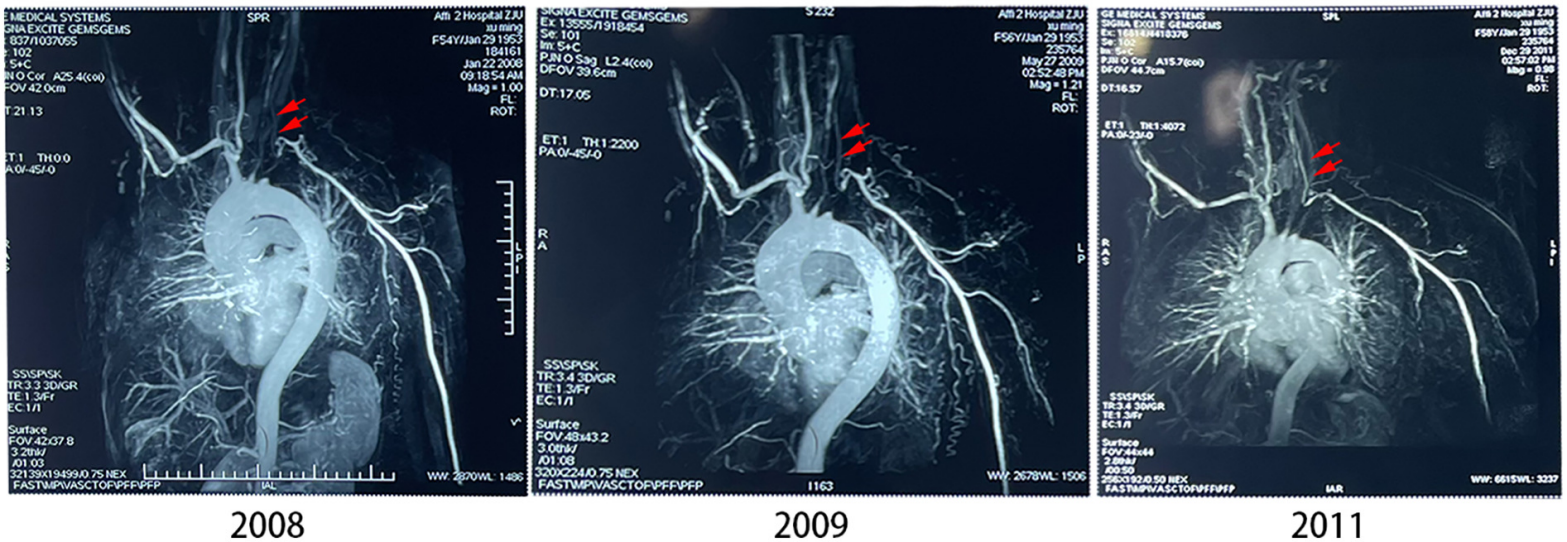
MRI of the aortic vasculature in three time points. Red arrows indicated the locations of the left common carotid artery in the subsequent different years after 2008.
